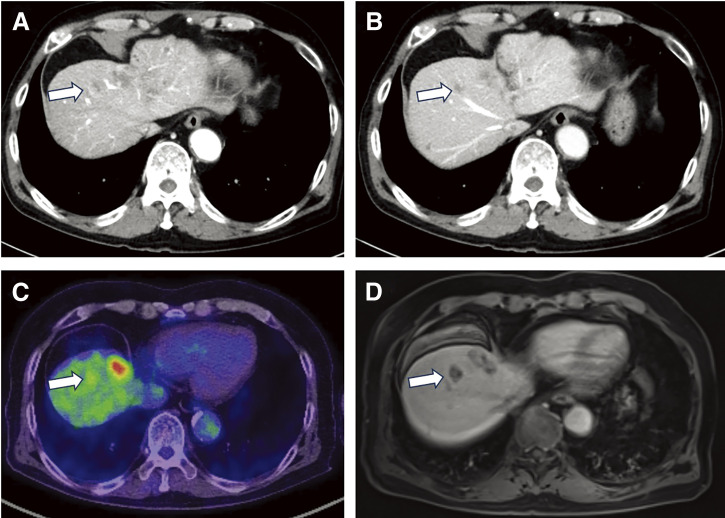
Preoperative images by CT, PET, and MRI. (A) CT (portal phase). (B) CT (equilibrium phase). (C) PET. (D) Ethoxybenzyl–magnetic resonance imaging (EOB-MRI). white arrow: new lesion.
